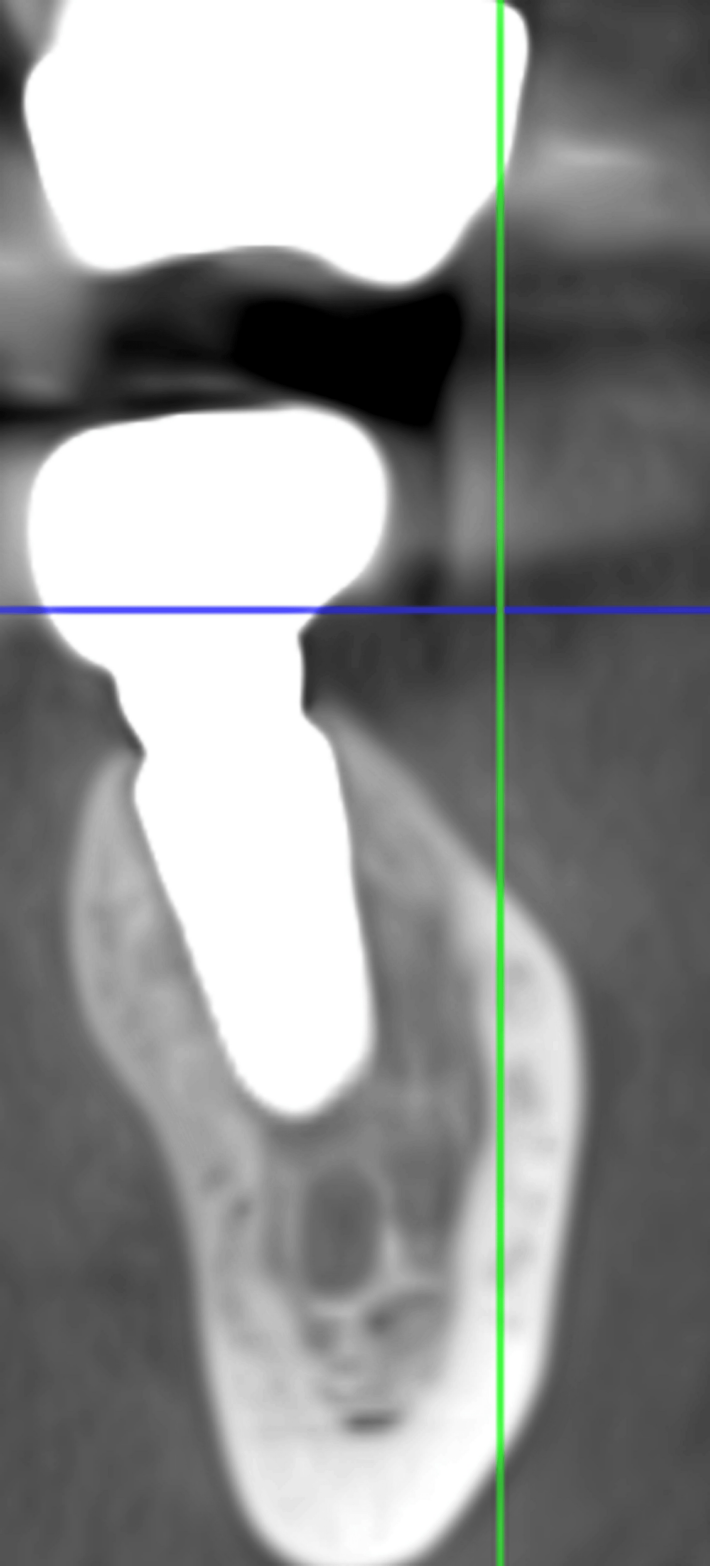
Cone beam computed tomography (CBCT) at three months
